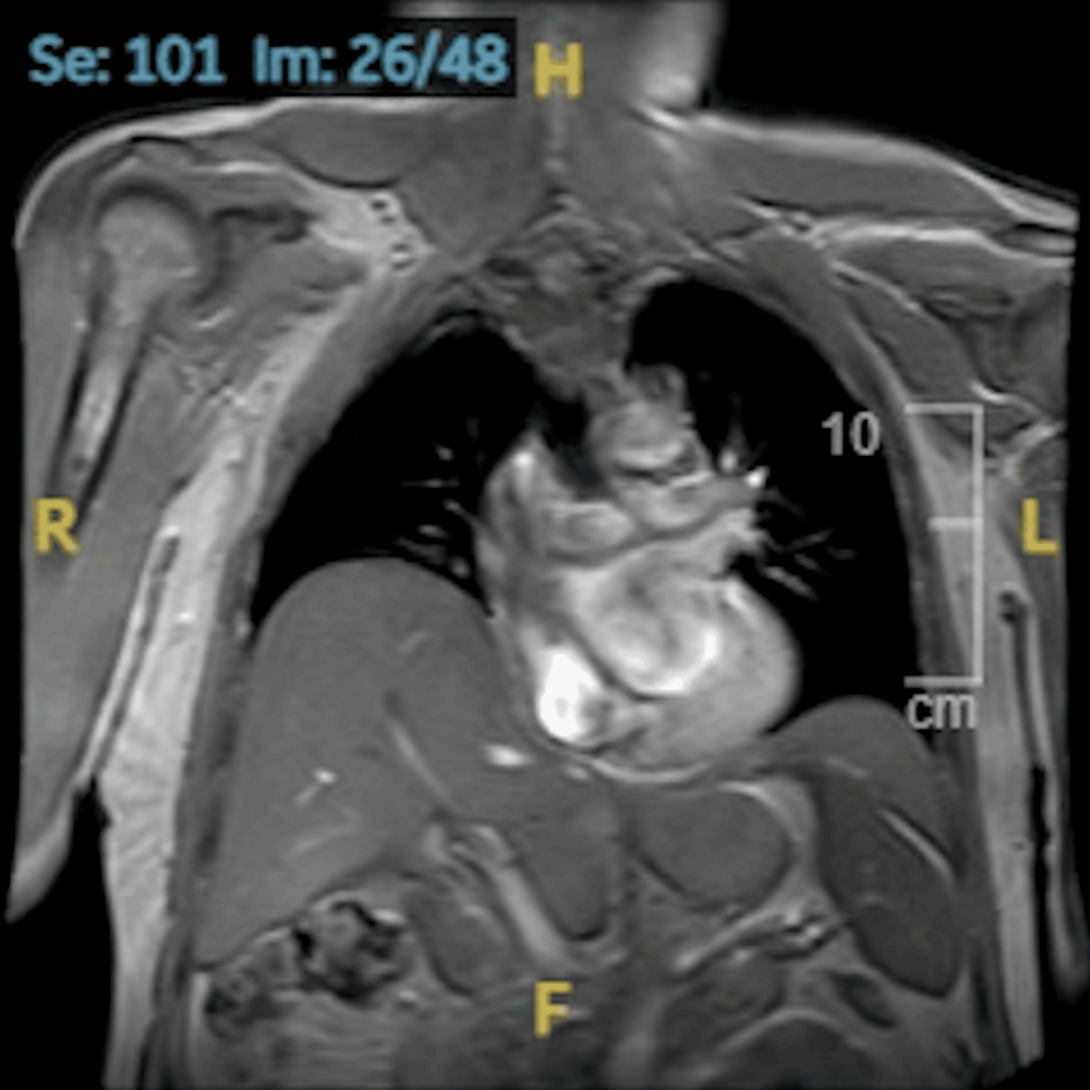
Cardiac MRI three days prior to ICD insertion The MRI image taken three days before ICD insertion shows a raised hemidiaphragm, looking very similar to the CXR image postoperative. Please note that the MRI scan was taken supine, while CXR was taken erect, which is a limitation of the comparison. However, the shape of the diaphragm and the vertical distance between the left and right diaphragm are quite similar ICD: implantable cardioverter defibrillator; MRI: magnetic resonance imaging; CXR: chest X-rays
